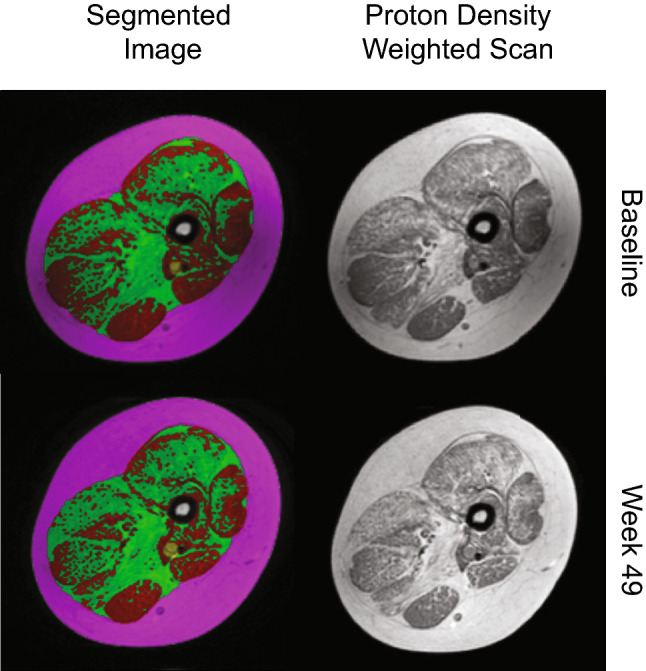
Representative example of a single slice of the thigh MRI at two time points. Representative image showing the proton density weighted scan at baseline and week 49. Both the acquired and segmented images are shown. Magenta region shows subcutaneous fat, red region show lean muscle, and green region shows inter/intramuscular fat. *MRI* magnetic resonance imaging
